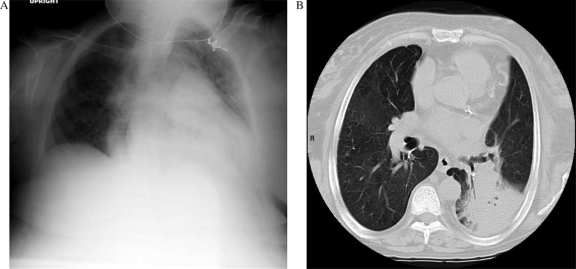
Chest X-ray (A) and CT scan (B) show left lobe infiltrates.
